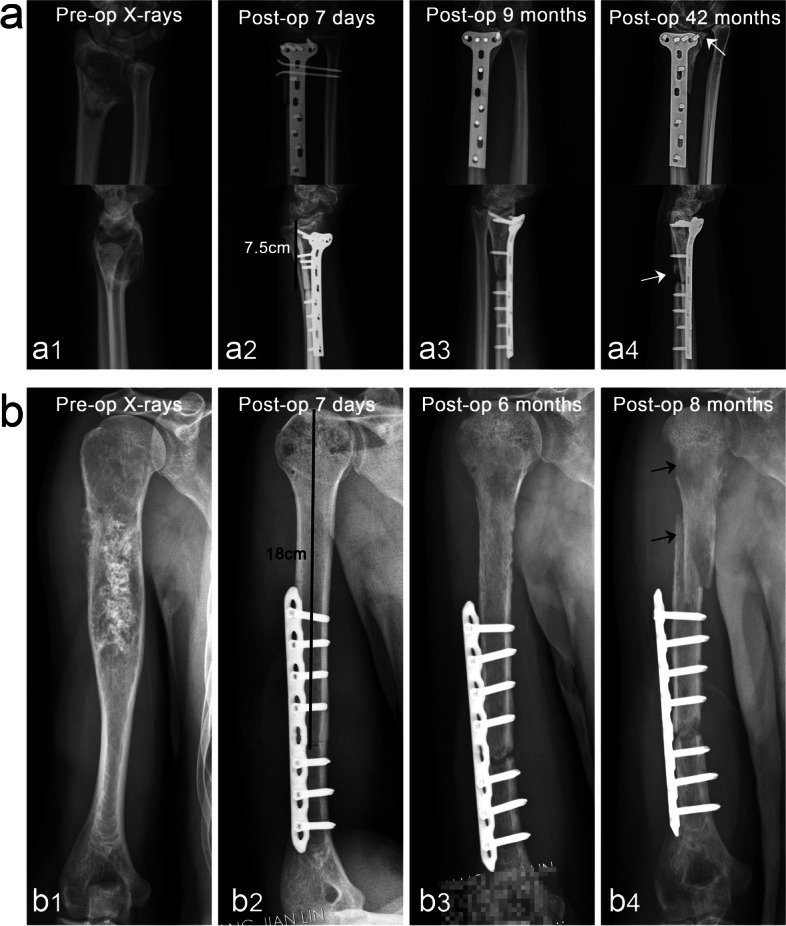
Complications of bone and joint allograft (cases 23 and 38). In the patients with stage IIB fibrosarcoma of distal radius, stage IIB chondrosarcoma of proximal humerus was treated with allograft bone joint transplantation to repair bone defects. a1 Preoperative anteroposterior and lateral X-rays. a2–a4 Continuous follow-up of X-ray after surgery, the proximal part of the allograft gradually developed bone resorption leading to bone nonunion, and the distal carpal surface developed secondary osteoarthritis (white arrows indicate sites of nonunion and osteoarthritis, respectively). b1 Preoperative anteroposterior X-rays. b2–b4 The length of the allograft was 18 cm. Follow-up revealed that the allograft was gradually absorbed, with nonunion of the host–donor junction, loosening of the internal fixation, and eventually the allograft fracture occurred (black arrows indicate sites of bone resorption and fracture location, respectively)
